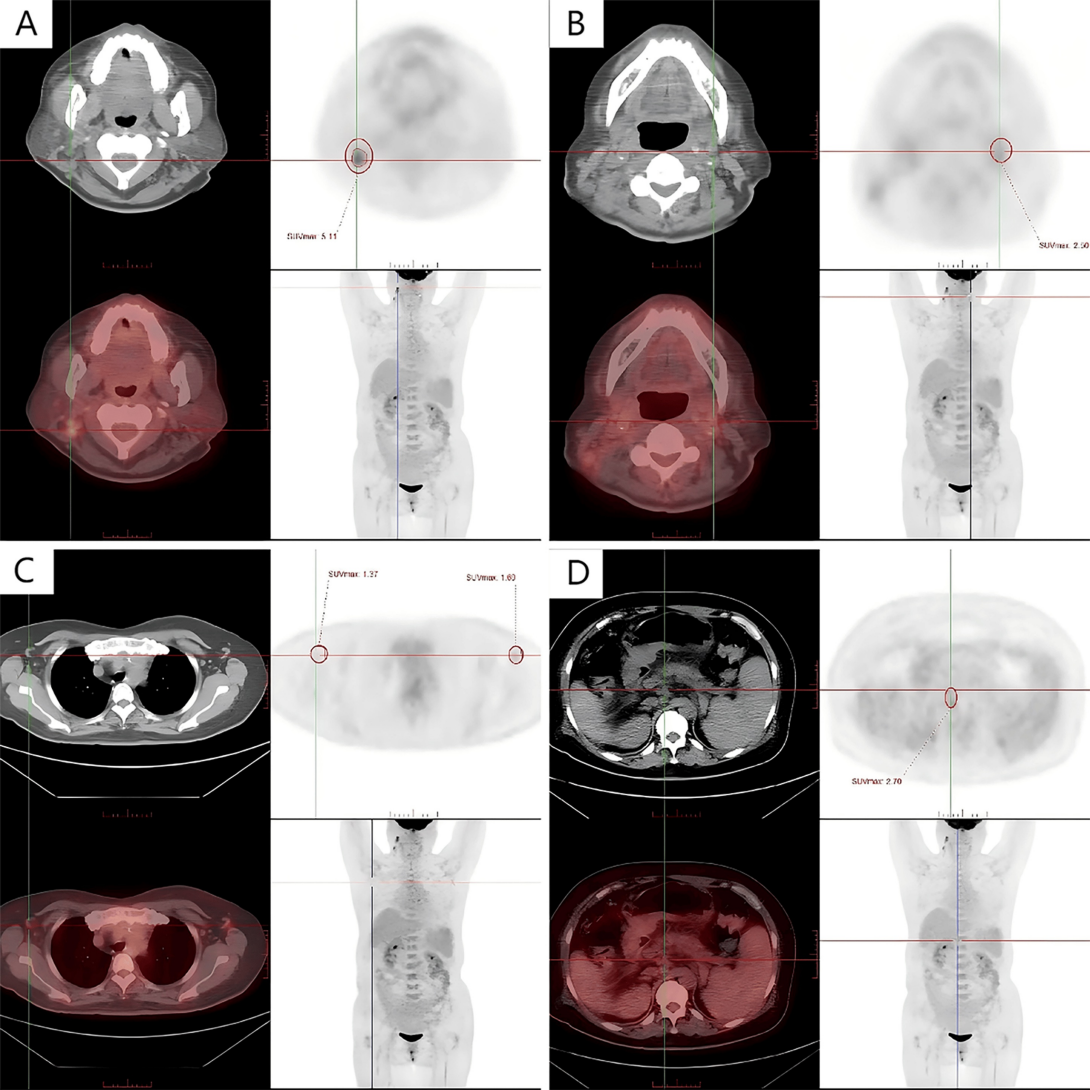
PET/CT images. (A) Multiple enlarged lymph nodes in region II of the right neck, larger about 1.5cm x 1.4cm, SUVmax=5.11 (red circle). (B) Multiple lymph node formations were seen in the left cervical region I-V, some with increased metabolism, SUVmax=5.11 (red circle). (C) Lymph nodes with slightly higher metabolism were seen in both axillae, the sizes were about 1.4cm x 0.8cm, SUVmax=1.37-1.69 (red circles). (D) Multiple lymph nodes were seen slightly enlarged in the hepatoportal region and hepatogastric ligament, some of them mildly metabolized, maximum about 1.2 cm x 0.9 cm, SUVmax=2.70 (red circle).
